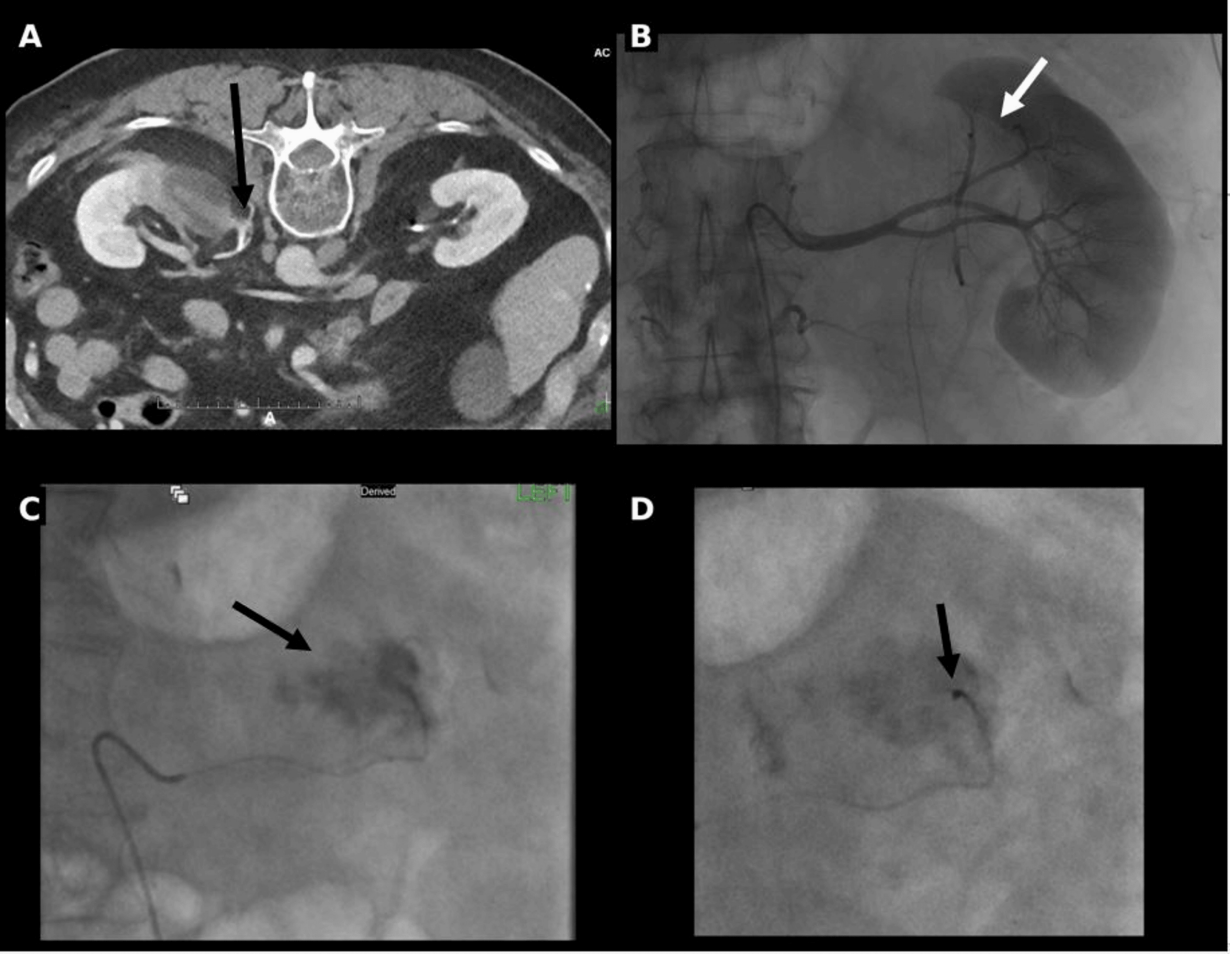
Angiographic evaluation and coil embolization of the post-ablation hemorrhage (A) Axial contrast-enhanced CT image obtained post-ablation, demonstrating a small perinephric hematoma and active extravasation (black arrow); (B) Selective left renal arteriogram demonstrating the ablation zone with the corresponding area of hypoenhancement (white arrow); (C) Superselective angiogram showing contrast extravasation from a distal upper pole segmental artery branch (black arrow); (D) Post-embolization angiogram following deployment of a 2 mm detachable coil (black arrow) within the culprit vessel, resulting in occlusion and resolution of the extravasation.
